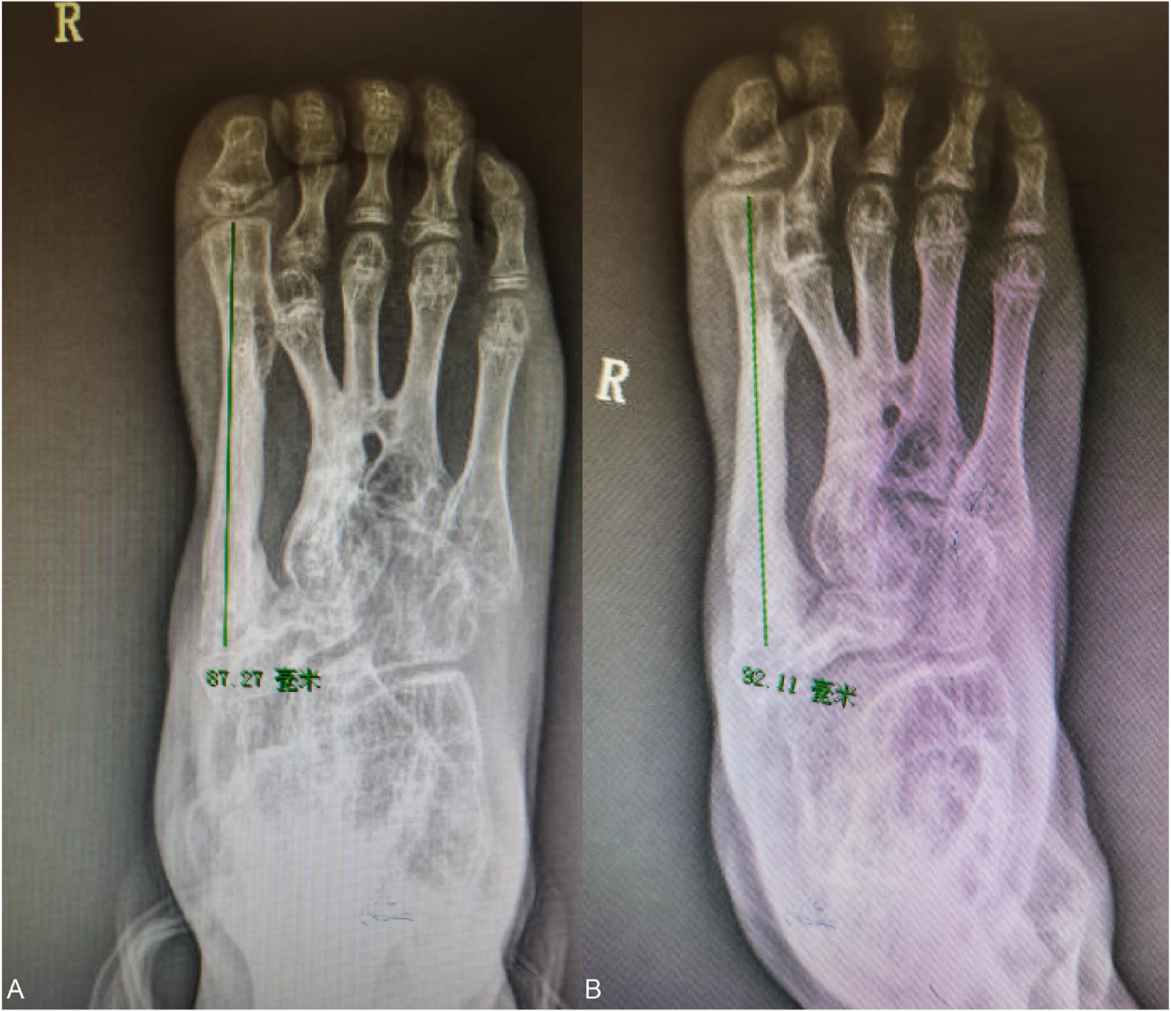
(A,B) comparison of grafted bone length in the second six months orthopantomogram (left) and eight months orthopantomogram (right): the first metatarsal train followed the patient's developmental growth by 5 mm.
